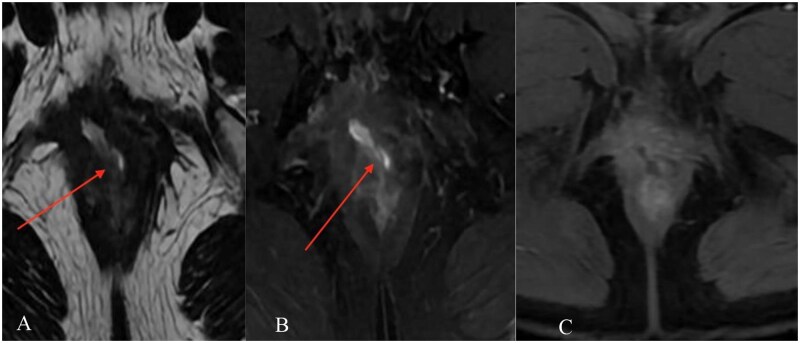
Primary transsphincteric fistula and internal opening on pelvic MRI. (A) Axial T2-weighted image shows the internal opening at approximately the 11 o’clock position (arrow). (B) Coronal fat-suppressed T2-weighted image demonstrates the fistulous tract extending anterolaterally to the right (arrow). (C) Coronal T1-weighted image provides an anatomic overview of the anorectal region and adjacent pelvic structures.
